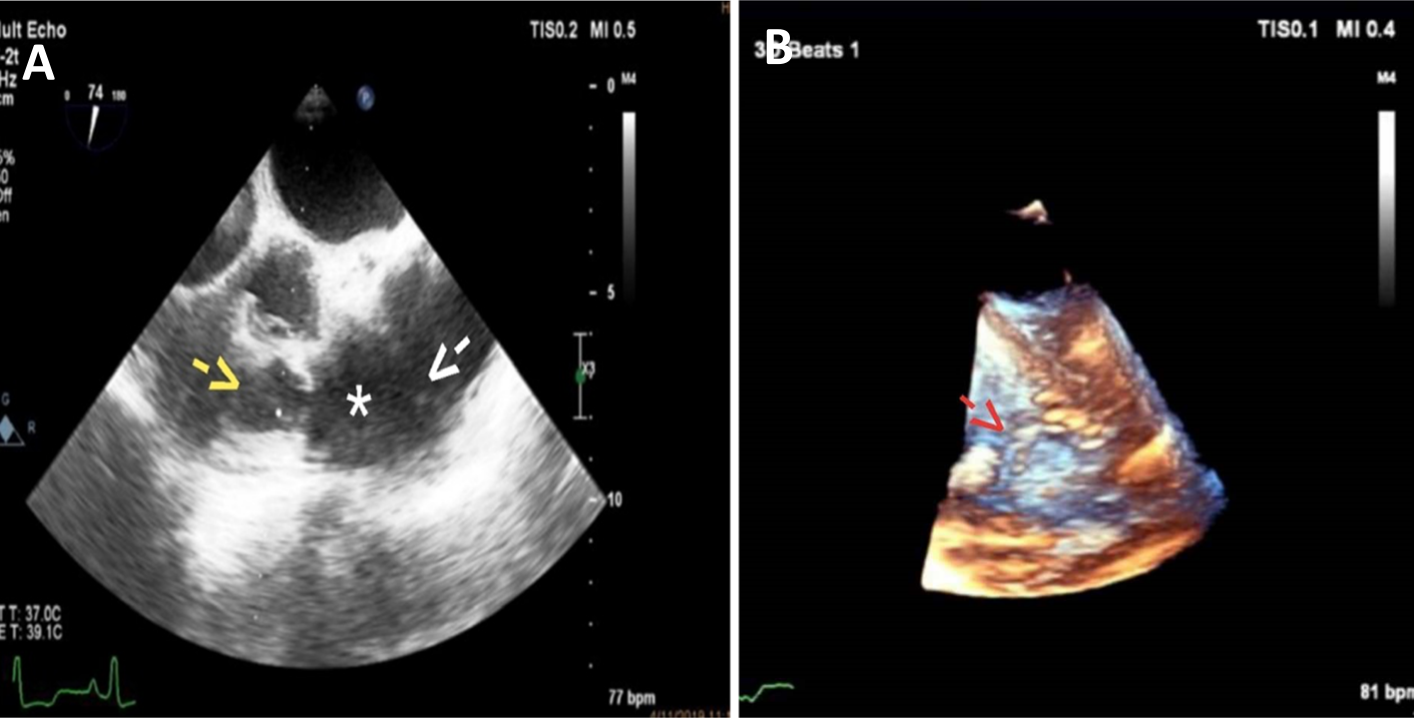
A- 2D Transesophageal echocardiography (TEE) of dome shaped pulmonic valve with vegetation (Yellow arrow) and post stenotic dilatation of pulmonary artery (*) and vegetation on pulmonary artery wall (Endarteritis) white arrow, 74 degree. B -3D TEE echoardiography of vegetation in main pulmonary artery wall (Red arrow)
